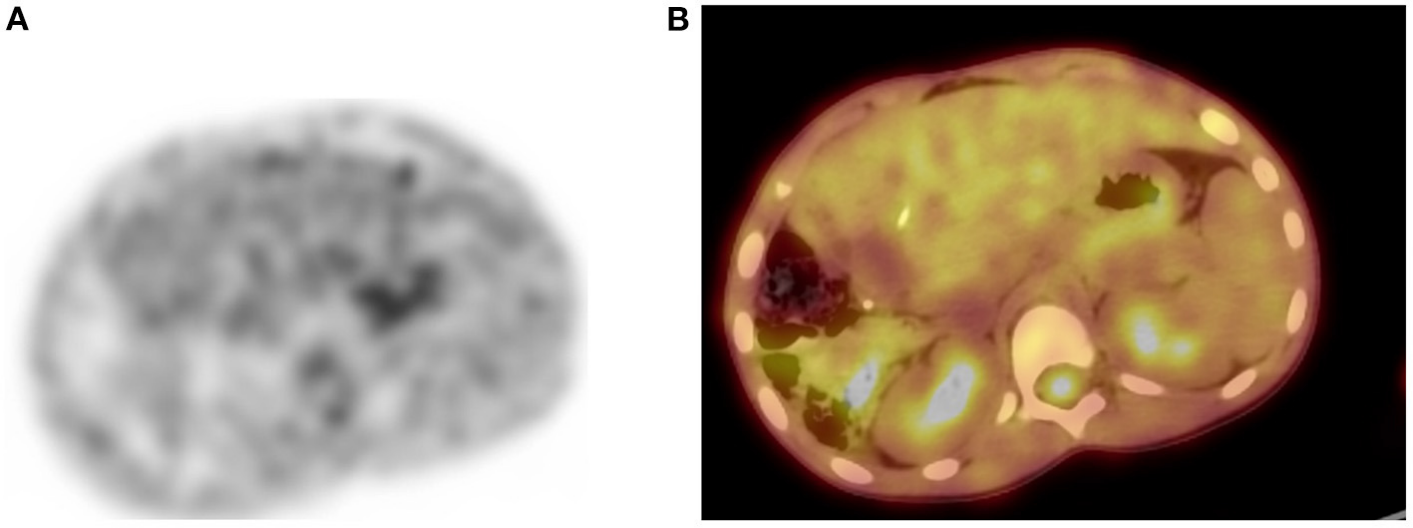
Diffuse gastric uptake. (A) FDG PET Axial and (B) combined PET/CT, showing diffuse uptake in inferior greater curvature of the stomach of a young patient who received a liver transplant. He continues to complain of abdominal pain following transplant procedure and all his inflammatory markers were raised. The visualized increased diffuse uptake was suggestive of gastritis and gastroscopy confirmed inflammation.
